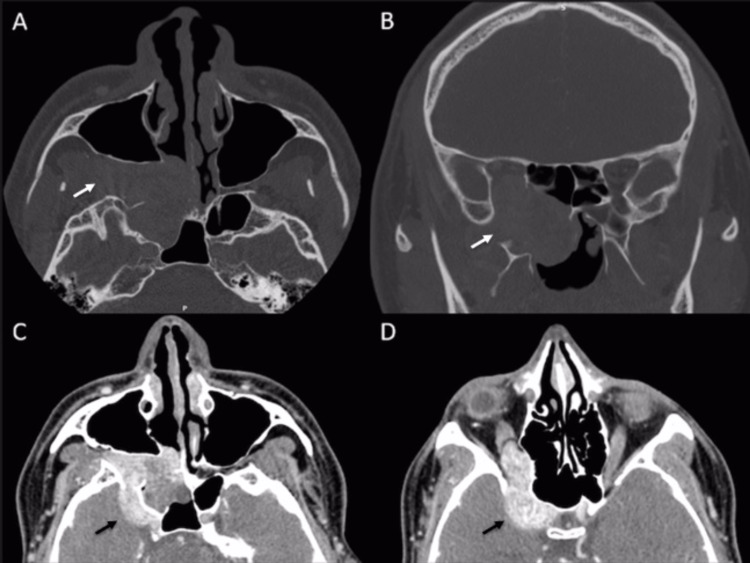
Preoperative sinonasal CT scan The arrows in 1A, 1B, 1C, and 1D depict a lobulated hypervascular lesion with remodeled adjacent bone structures, an enlarged sphenopalatine fissure and pterygomandibular fossa, and an enlarged right foramen rotundum and inferior orbital fissure with extension to the posterior regions of the right orbital fossa. This lesion extends medially and inferiorly toward the nasal fossa. CT: computed tomography
